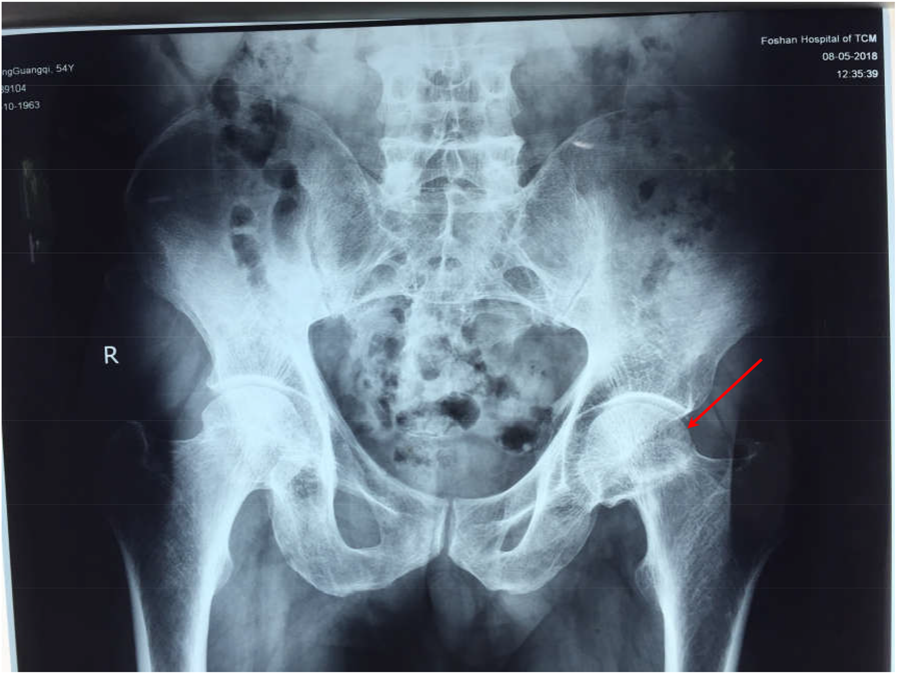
Plain radiograph of the pelvis indicating old fracture of the left femoral neck (red arrow) at a hospital in Foshan, Guangdong Province
